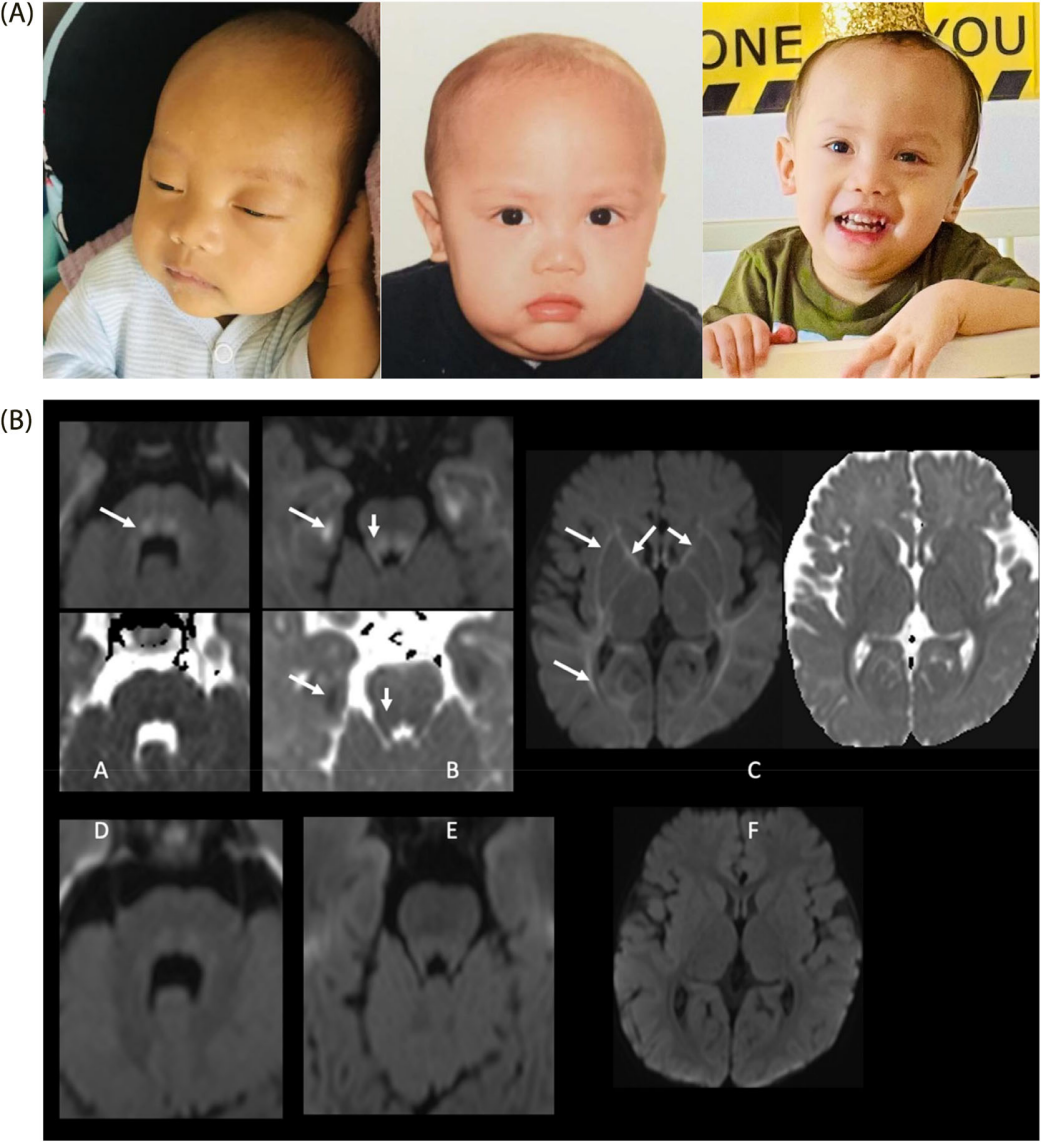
Dysmorphic features and brain MRI findings. A) Picture on the far left shows our patient at 3 months of age with jaundice, frontal bossing, macrocephaly and coarse face. Picture in the middle shows our patient at 11 months of age after initiation of low‐methionine diet. We can see the same dysmorphic features in addition to body‐built improvement following weight gain. Picture on the right is his second birthday, hypotonia can be appreciated in the hands. B), Brain MRI finding showing diffusion imaging (b = 1000) and ADC map at 6 (A, B, C) and 12 (D, E, F) months of age. Panel A showed descending dorsal fibers of the tegmentum with high signal (white arrow) that resolved at 12 months of age (Panel D). Panel B shows restricted diffusion in the mesial temporal subcortical white matter as well as in the tegmentum and superior cerebellar peduncles (white arrows) that resolved at 12 months of age (Panel E). Panel C shows mild restricted diffusion in the supratentorial white matter involving the internal and external capsules, optic radiations and subcortical white matter (white arrows) that resolved at 12 months of age (Panel F)
